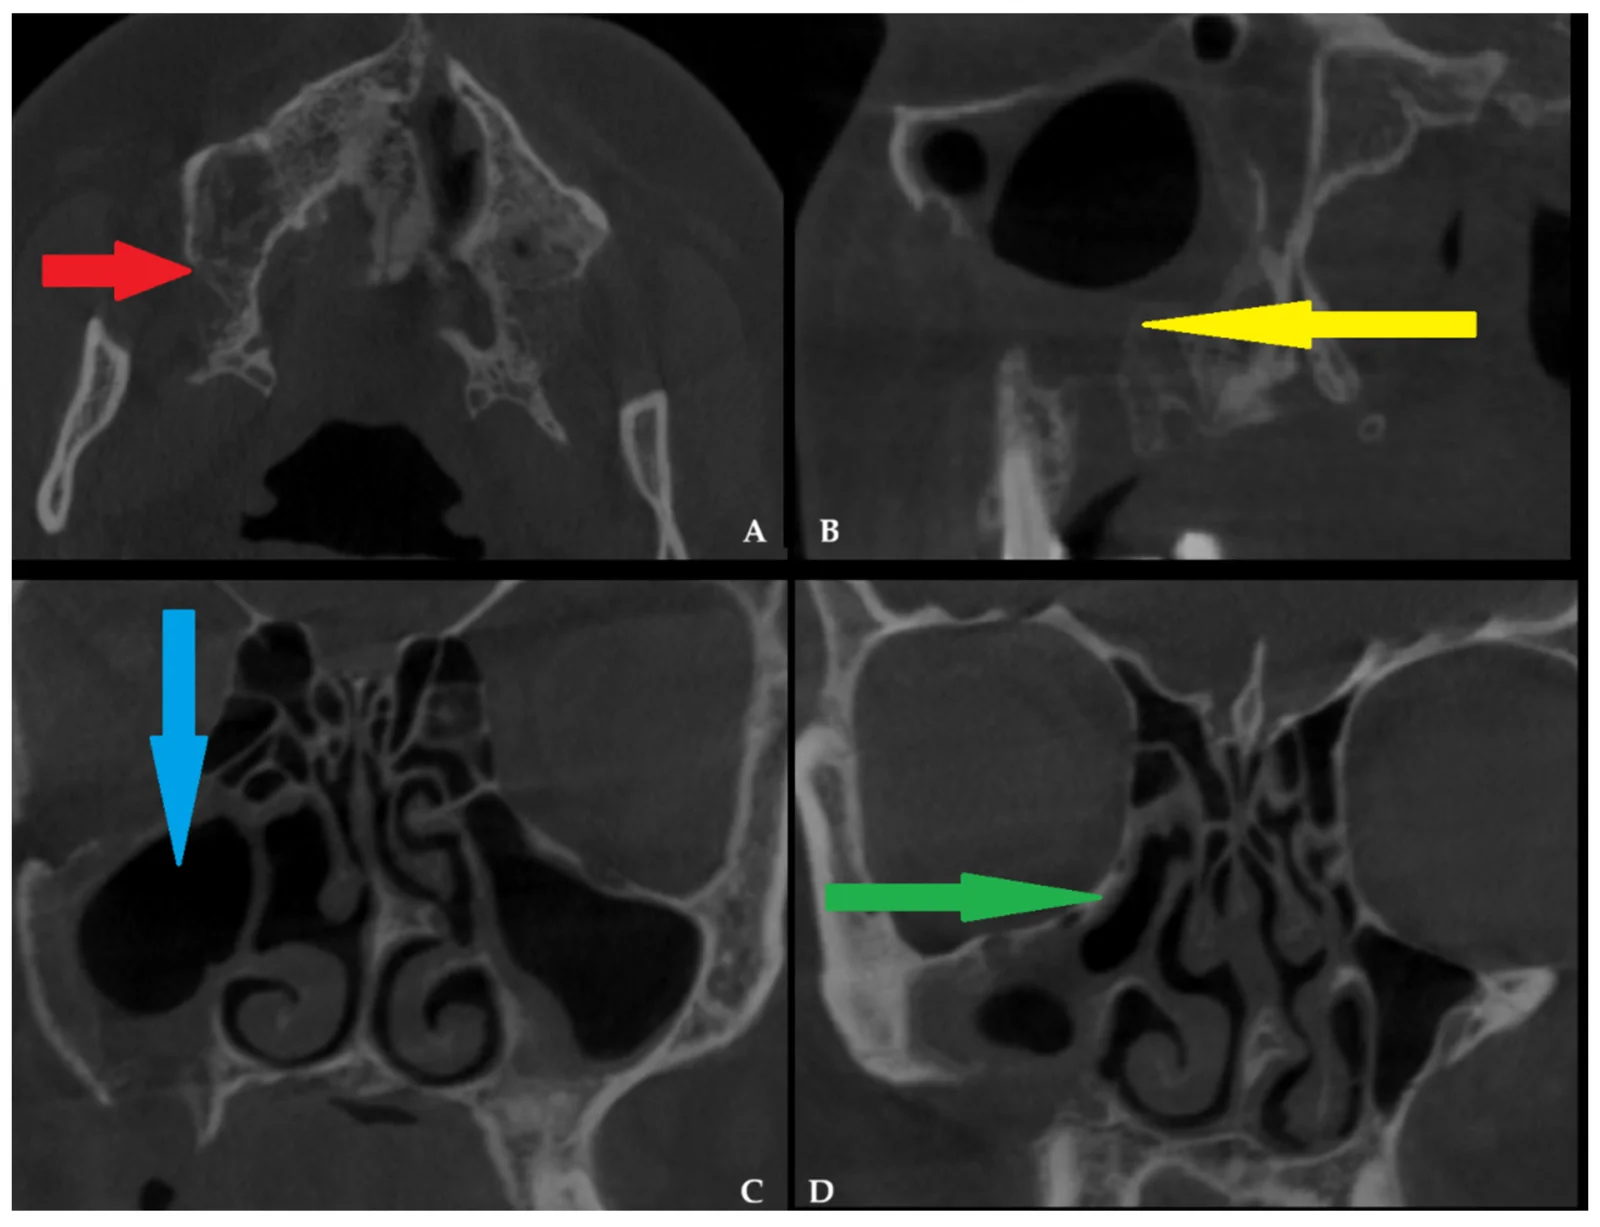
Early postoperative results at 3 months revealed very good and satisfying results visible in a CBCT (cone beam computed tomography) study (20 × 20 FOV (field of view); imaging protocol based on RayScan S 5471.3 mGy, RayCompany Ltd., Samsung 1-ro, Hwaseong-si, Gyeonggi-do, Republic of Korea). Although traditional and less frequently utilized today, the CLA remains highly valuable for managing the maxillary sinus. When combined with simultaneous tooth extraction, the complete curettage/removal of periapical lesions, and bone ostectomy ((A,B), red and yellow arrows), this approach facilitates thorough sinus irrigation ((C), blue arrow). Furthermore, establishing dual sinus drainage consisting of OMC irrigation with curettage ((D), green arrow) and an inferior nasal antrostomy not only restores sinus ventilation but also eliminates progressive inflammatory processes, effectively halting the recurrence and spread of ODS. Such an approach should take into consideration the presence of active tooth-related periapical lesions, their further possible spread into the sinus, and a few episodes of ODS exacerbation [19,20,21,22,23,24,25]. On the other hand, the timing of this procedure should consider the time without any active soft tissue inflammation/purulent formation and the simultaneous combination of all of the mentioned bone, tooth, sinus, and nasal procedures to improve the patient’s condition and prepare the patient for any typical ODS treatment with FESS/ESS and/or dental treatment when necessary. This early satisfactory outcome was achieved when the simultaneous pharmacological therapy was intense in both pre- and postsurgical periods, while the single surgical approaches focused on removing teeth and dental- and sinus-related factors at once [25,26,27,28,29,30]. In this case, the otolaryngologist, based on worrisome nasal and sinus problems, improved the diagnostic accuracy with CT to fully visualize the scope of sinonasal problems. Furthermore, the intraoral examination revealed fully healed flaps with no evidence of an oroantral fistula, while the endoscopic evaluation showed no purulent discharge within the nasal cavity. The patient reported improved nasal breathing, reduced nasal fetor, and the complete resolution of sinus pain. The 3-month CBCT showed stable sinus aeration without recurrent opacification, while endoscopic examination showed no purulent discharge; no clinical recurrence was observed at 12 months.
